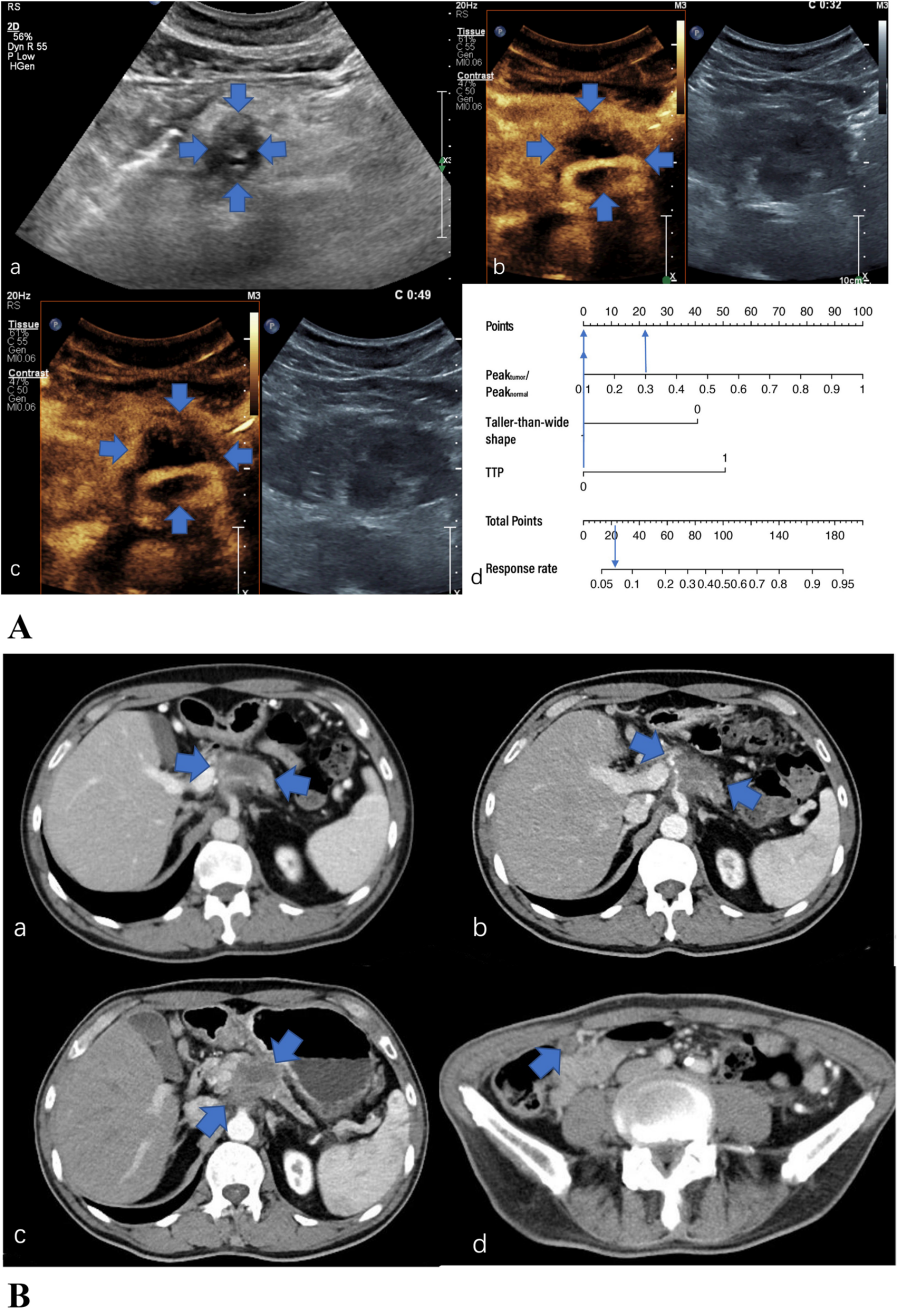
A US and CEUS of a 63-year-old man with a 4.1 cm hypoechoic solid lesion in the head of the pancreas. a The lesion was taller-than-wide in the longitudinal plane. b The TTP was 32 s and the Peak_tumor_/Peak_normal_ was 0.306 (in the transverse plane). c The lesion in the venous phase(in the transverse plane). d A total of 22 points were assigned to the lesion according to the nomogram, corresponding to an about 7% probability of “responders”. After 6 cycles of NAC, a radiographic response of PD (metastasis to paracolic lymph nodes) achieved. B The pre-treatment (a) and post-treatment (b, c, d) CT images of the case. The tumor was larger (b, c) and there was suspected paracolic lymph node metastasis (d) after 6 cycles of NAC.CA 19–9 decreased from 177.2 to 76.7U/mL
